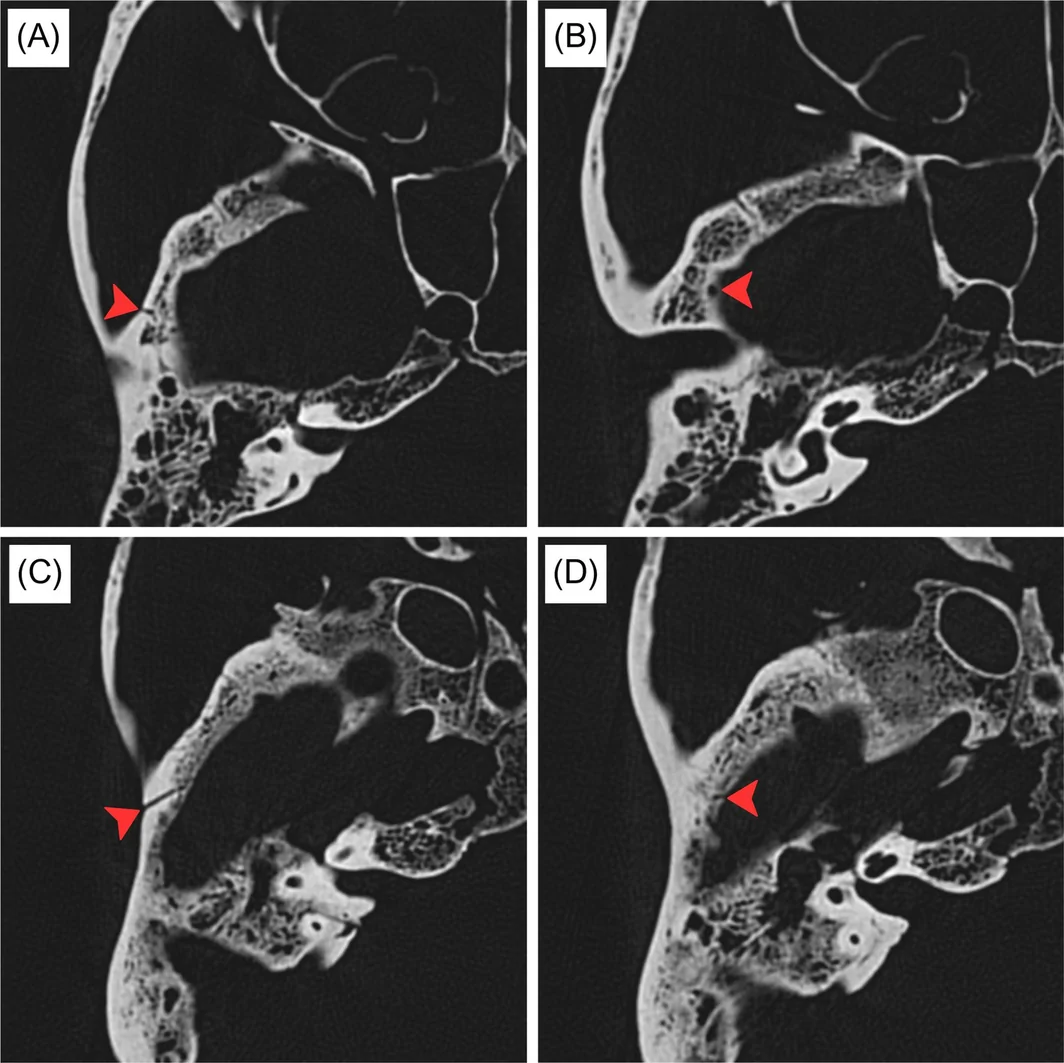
Axial computed tomography images showing the anterior type (A, B) and posterior type (C, D) of the foramen of the squamous part of the temporal bone. The opening from the outer surface of the temporal bone (A, C) and the corresponding opening from the inner surface (B, D) are indicated by the red arrowheads.
